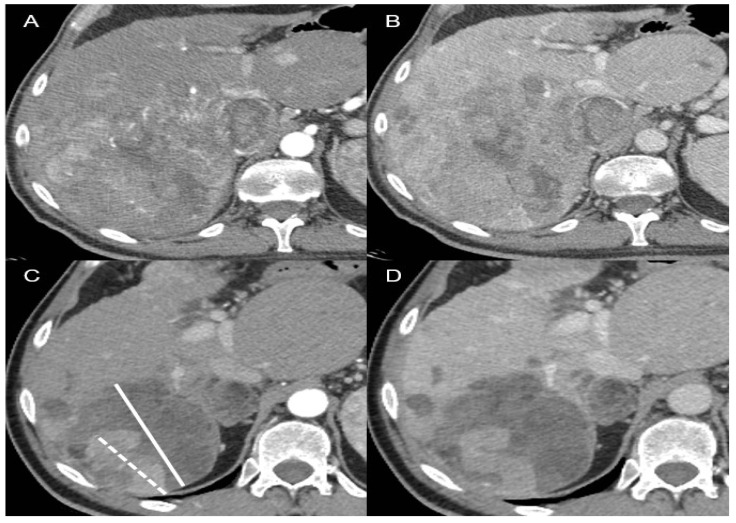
A 61-year-old man with infiltrative hepatocellular carcinoma and tumour thrombosis of the inferior vena cava: On baseline contrast-enhanced CT ((A), arterial phase; (B), portal venous phase), the largest tumour diameter measures 12.8 cm. CT follow-up ((C), arterial phase; (D), portal venous phase) after three months of atezolizumab–bevacizumab demonstrates a decrease in the size of the lesion, with a total tumour diameter of 10.8 cm (continuous line), consistent with stable disease according to the RECIST 1.1 criteria, but with a residual internal enhancing component of 6.1 cm (dashed line), consistent with a partial response according to the mRECIST criteria.
